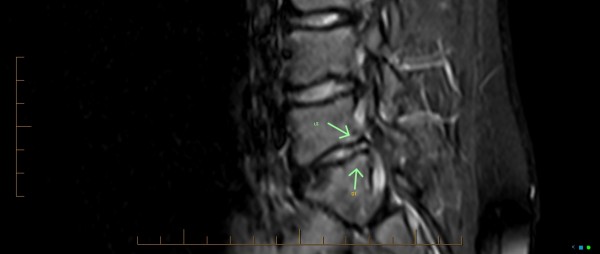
MRI, sagittal STIR view. The L5/S1 subchondral bone marrow hyperintensity (areas of white) seen on both sides of the L5/S1 disc (arrows), is suggestive of annular injury and ongoing inflammatory response. L4/5 right paramedian disc protrusion, and L5/S1 disc height reduction with dehydration and right posterolateral disc protrusion that appears chronic. Anterior sacral base bone marrow hypointensity indicates chronic marrow repair.
